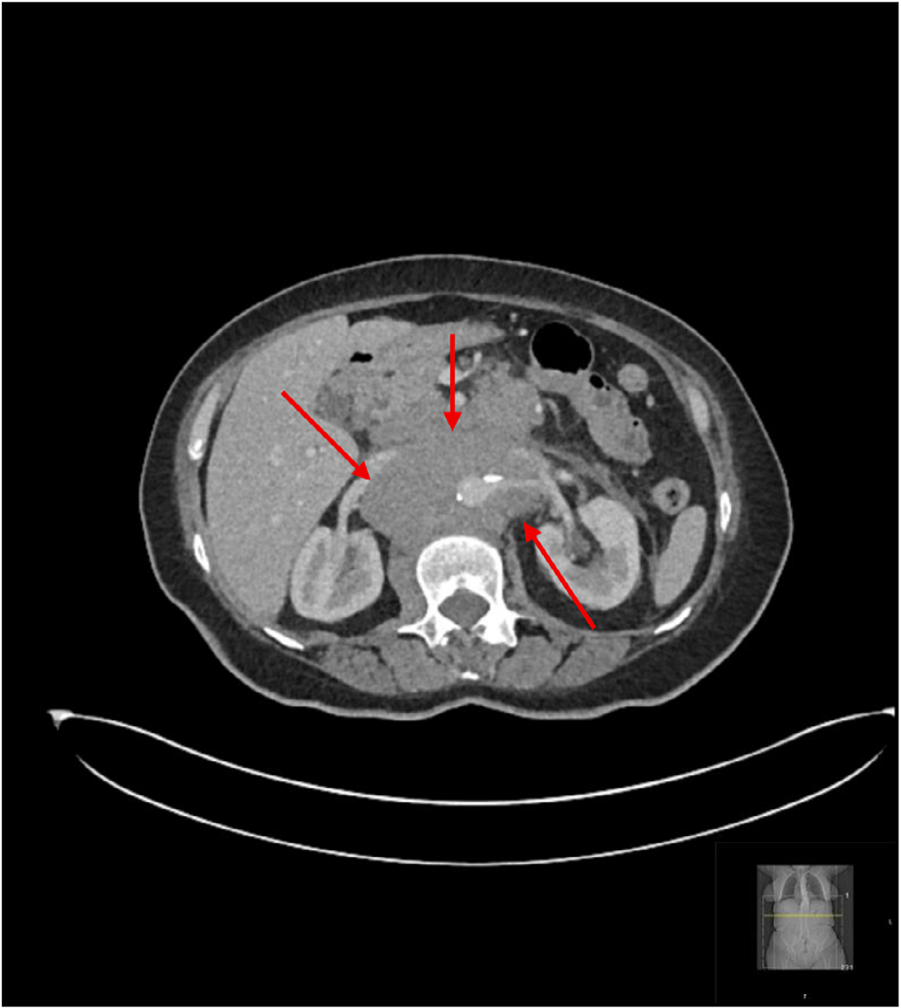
CT scan of the abdomen showing retroperitoneal lymphadenopathy (red arrows).
